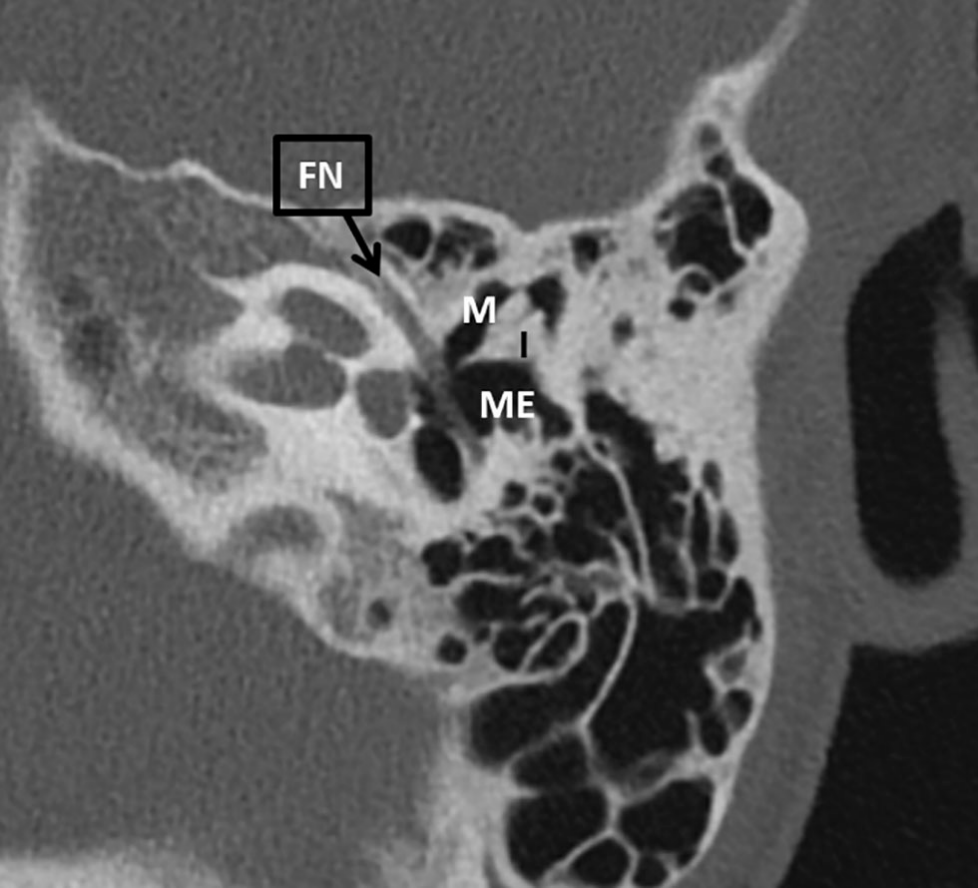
An axial CT scan of the left mastoid bone of patient 2. There is an epitympanic fixation involving the malleus. FN, facial nerve; I, incus; M, malleus; ME, middle ear.
